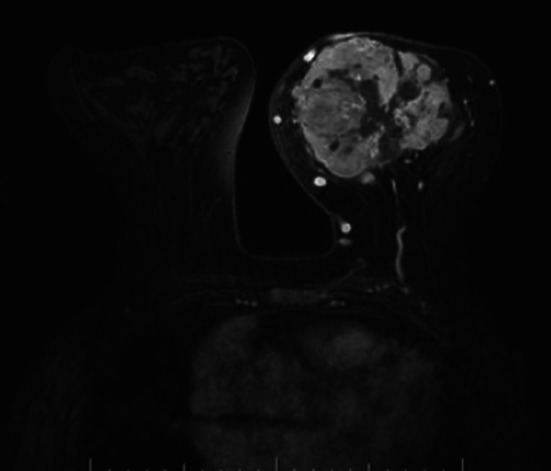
Contrast-enhanced magnetic resonance imaging revealing a large lobulated mass. No apparent infiltration to the pectoral major muscle can be observed
